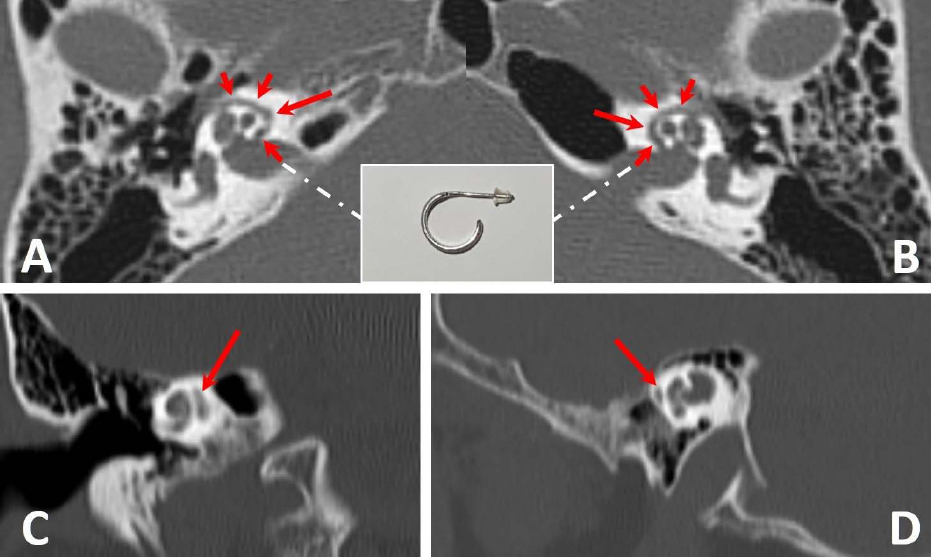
A 70-year-old woman with incidental advanced pericochlear otosclerosis showing the C-hoop earring sign. Sinus CT images on bone algorithm and windows show (A) right, and (B) left magnified axial images of the temporal bones at mid-cochlea levels with bilateral, symmetric, well-marginated, curvilinear tubular-like (1.1 mm in diameter) lucencies (red arrows) within the otic capsules, extending on each side from the middle ear cavity just anterior to the oval window anterolaterally, to the internal auditory canal posteromedially, and around the cochlea, consistent with advanced pericochlear (retro-fenestral) otosclerosis. No otospongiotic changes are present. The inset image shows the analogous C-hoop earring. (C) and (D) show magnified coronal and sagittal images of the right and left temporal bones, respectively, at mid-cochlea levels with lucencies (red arrows) representing slices through the “C-hoop earring” of pericochlear otosclerosis.
